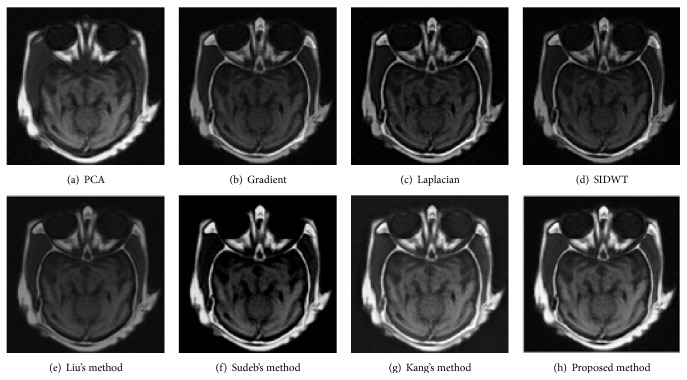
The fusion results of CT and MRI.
